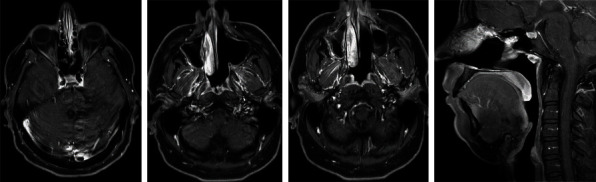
T1 post contrast fat sat axial (1st three) and sagittal (last one) MRI images showed complete resolution of the previous nasoethmoidal and nasochoanal/postnasal masses posttreatment (1st image is at the level of the ethmoid air cells, and rest of images are including the nasal choana and postnasal space).
